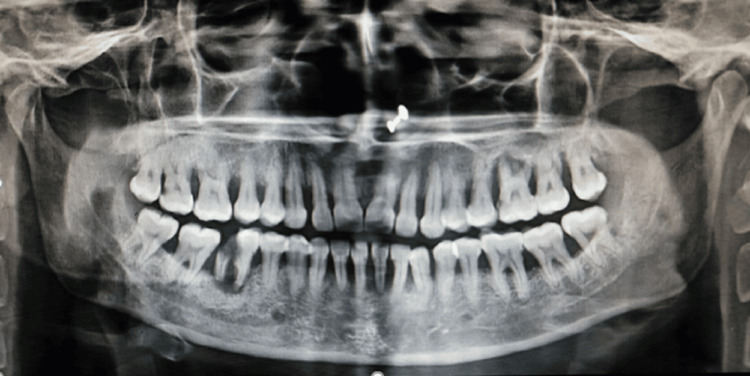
Orthopantomogram showing bone loss in the maxilla and mandible
